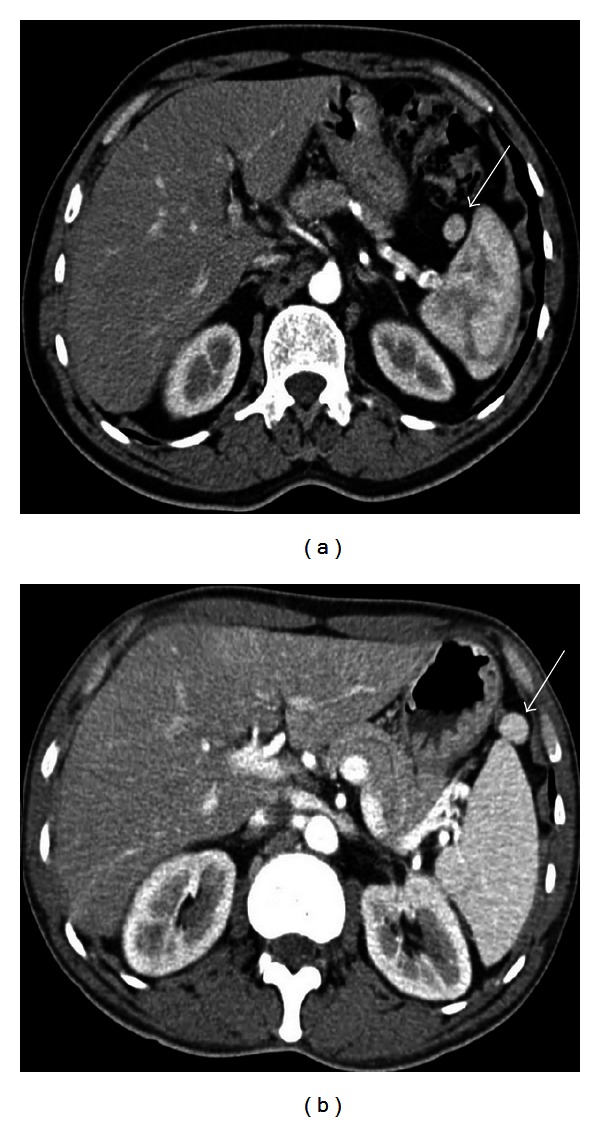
Axial contrast enhanced CT images (a), (b) of a 54-year-old male patient show wandering accessory spleen (arrow) anterior to the spleen which is at different locations in consecutive examinations.
